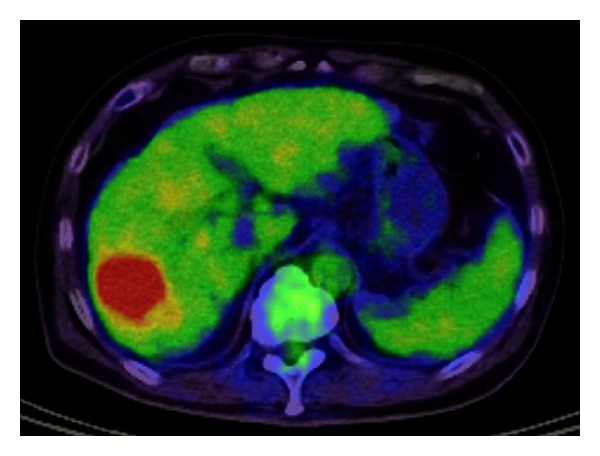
Positron emission tomography- (PET-) CT. Fluorine-18 fluorodeoxyglucose positron emission tomography (FDG-PET) shows abnormal uptake in segmental 6. The arrowhead denotes abnormal uptake lesion.
